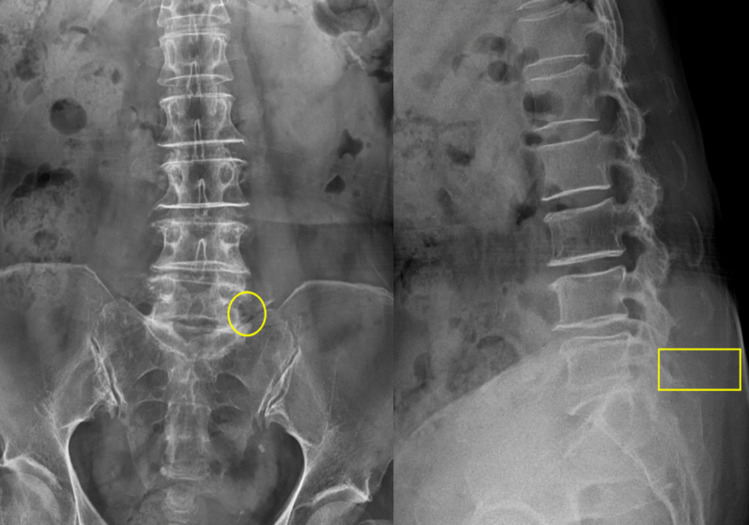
AP and lateral x-ray images. A AP view showing the yellow circle representing the position of an ideally placed Metrx tube with the inferolateral margin of the pedicle, the TP, the SAP, and the sacral ala are all included within its field. B In the lateral view, the Metrx tube should be inserted in alignment with the yellow box, ensuring that both the lower portion of L5 and the superior corner of S1 are visible within the tube’s field of view
